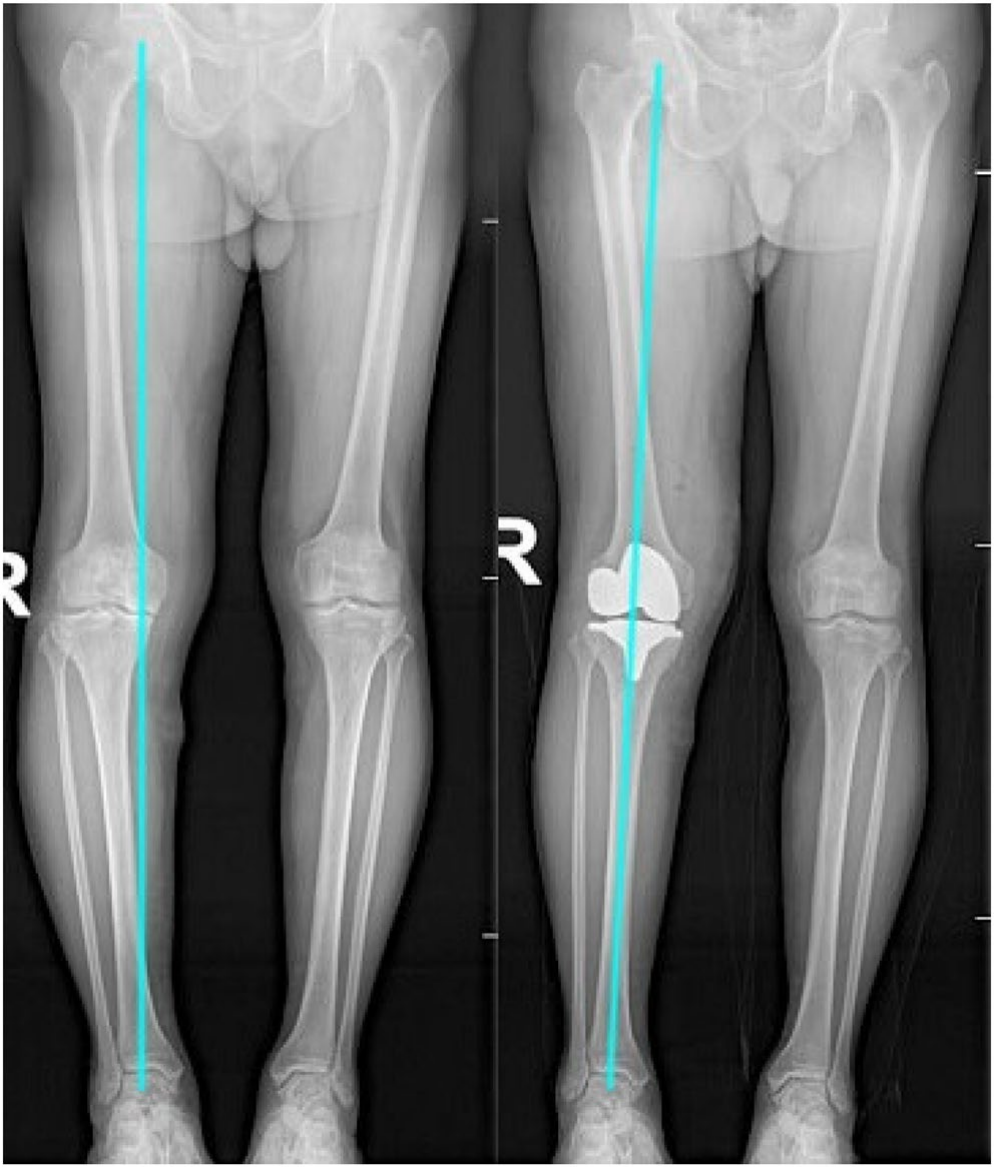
Postoperative long‐leg radiographs show that MA‐TKA changed the constitutional varus alignment to neutral. MA‐TKA, mechanical alignment‐total knee arthroplasty.
